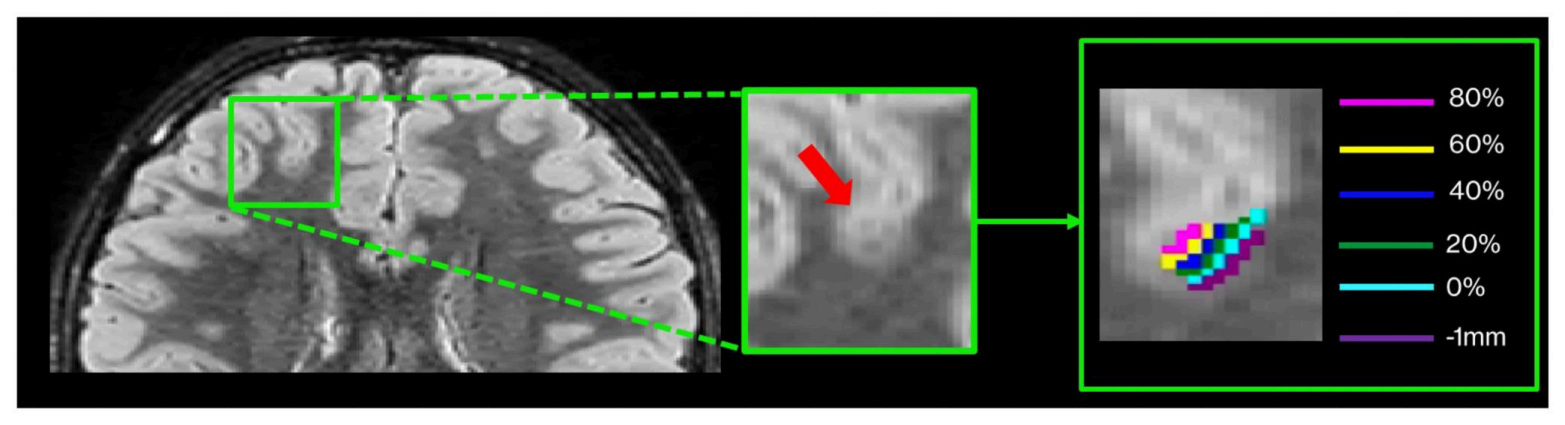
Surface-based quantitative magnetic resonance imaging profiles of focal cortical dysplasias (FCDs). Lesions were delineated on the fluid-attenuated inversion recovery images of each of the five patients with FCDs. The lesion masks were then mapped to each cortical depth in patients and controls in standard surface space. The mean and SD of qT1 and qT2 were extracted within the masks, after removing the effect of age and sex using linear regression. This allowed computation of the *z*-score profile of each patient normed against the controls, corrected for age and sex.
